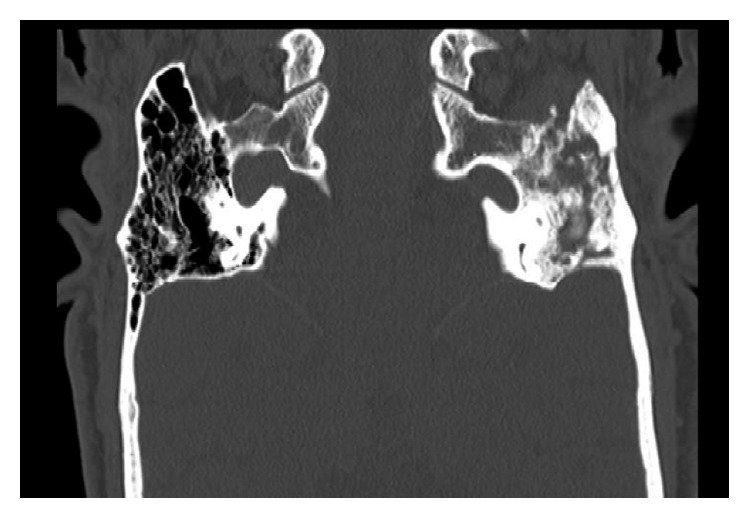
High-resolution CT scan (coronal cut) of the temporal bone. Total opacification of the left-sided hypo- and mesotympanum by a soft tissue density. Mastoid air cells totally opacified with resorption of septae and partially sclerosed bone. Partial dehiscence of the mastoid portion of the facial nerve canal. The left tympanic membrane is thickened and retracted inwards. The left middle ear ossicles and scutum are intact. Normal aeration of the external auditory canals with intact bony and cartilaginous boundaries. The right middle ear cavity is normally aerated with intact ossicles. The right mastoid air cells are unremarkable.
